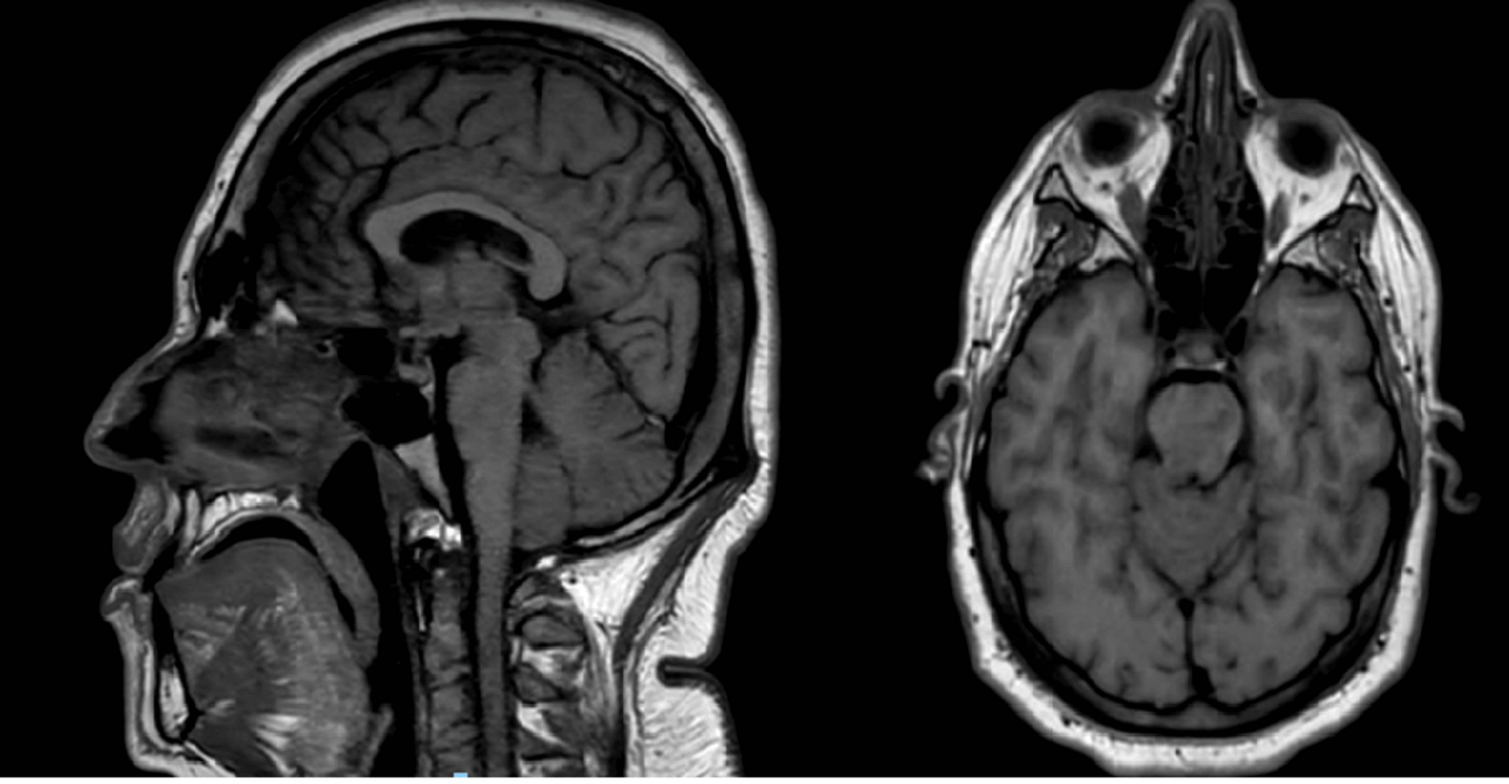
Brain MRI demonstrating pituitary stalk thickening up to approximately 4 mm, preservation of the optic chiasm, and absence of the normal posterior pituitary bright spot, suggestive of hypophysitis
